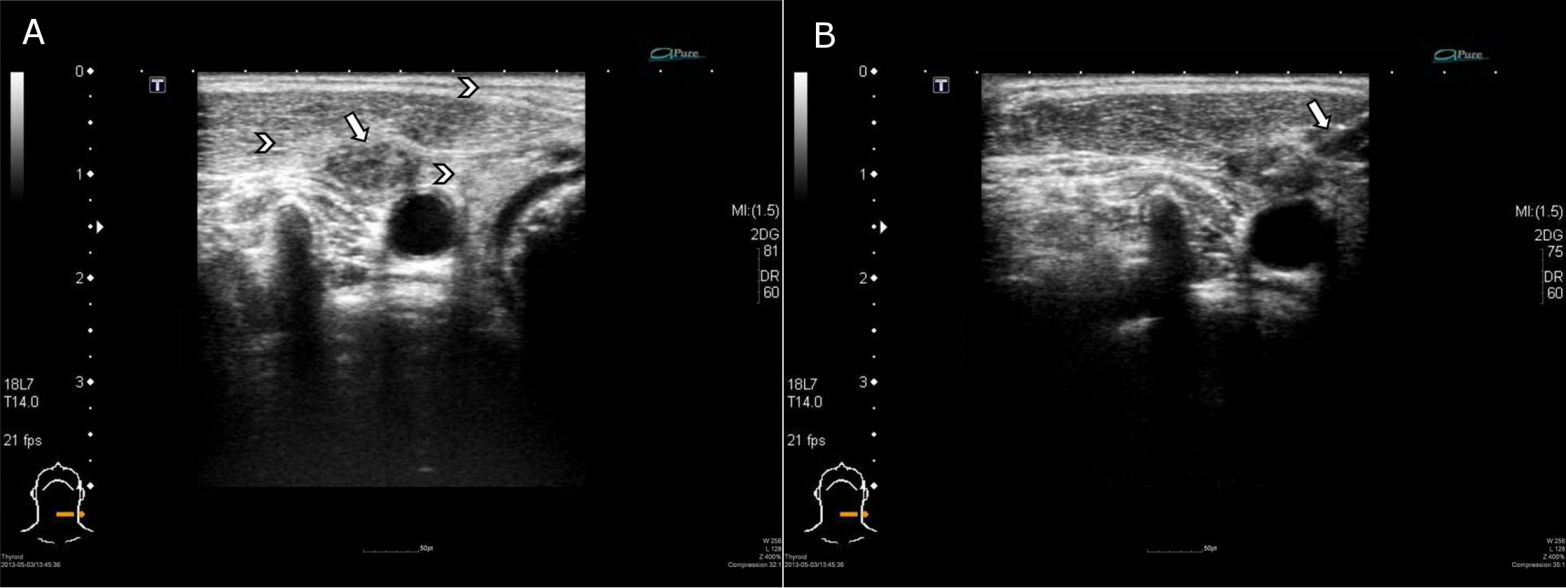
A 54-year old man who had a recurrent neck LN at left level IV after neck irradiation. (A)The left side ultrasound image showed that this metastatic LN was small, with an irregular margin and had no detectable calcification. Increased echogenicity of subcutaneous layer, overlying muscle and perinodal area was also noted. (arrow indicated irregular boundary and arrowheads showed increased echogenicity) (B)The right side image shows the USgFNA of the node. (arrow designated the needle)
